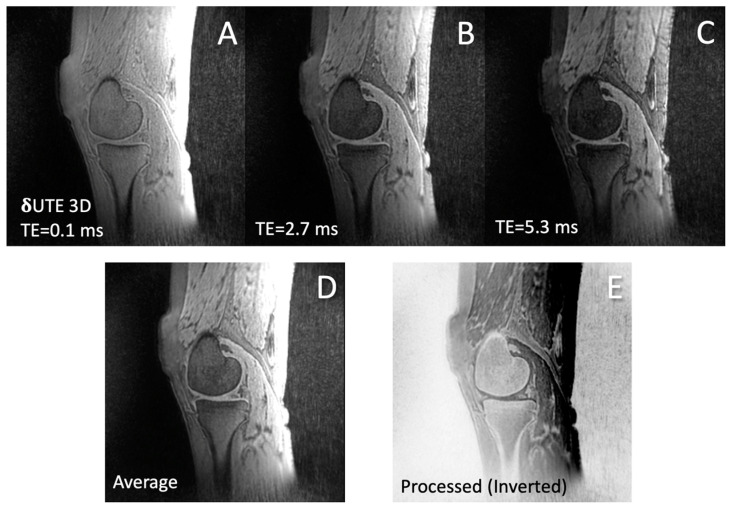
Image processing procedure. Source images from varying TEs (A–C) were first averaged (D) and then inverted (E) to create the final processed image with CT-like contrast for bone.
